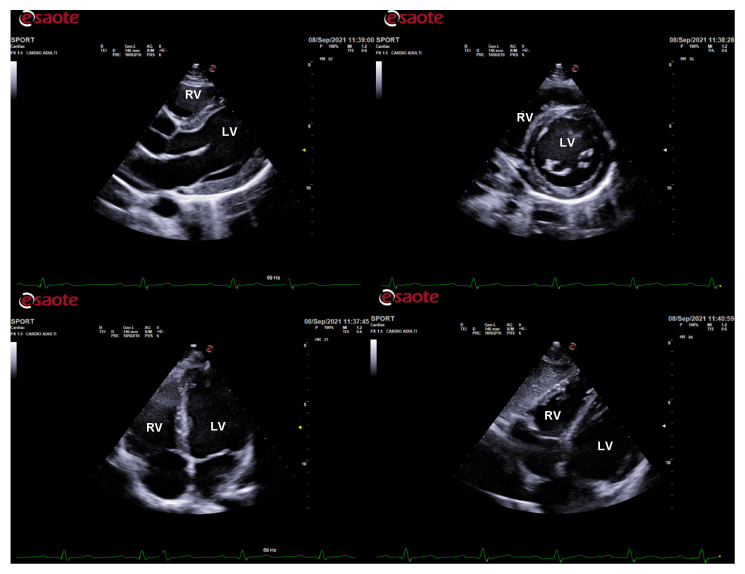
Standard echocardiography in an endurance athlete (cyclist). The physiological hypertrophy of the athlete’s heart is characterized by harmonic and symmetric wall thickening which is homogeneously distributed and involves all the cardiac chambers. In parasternal long-axis (top left), short-axis (top right), apical 4-chamber (bottom left), and subcostal (bottom right) views, both left and right chamber dilatation is evident. LV: left ventricle; RV: right ventricle.
